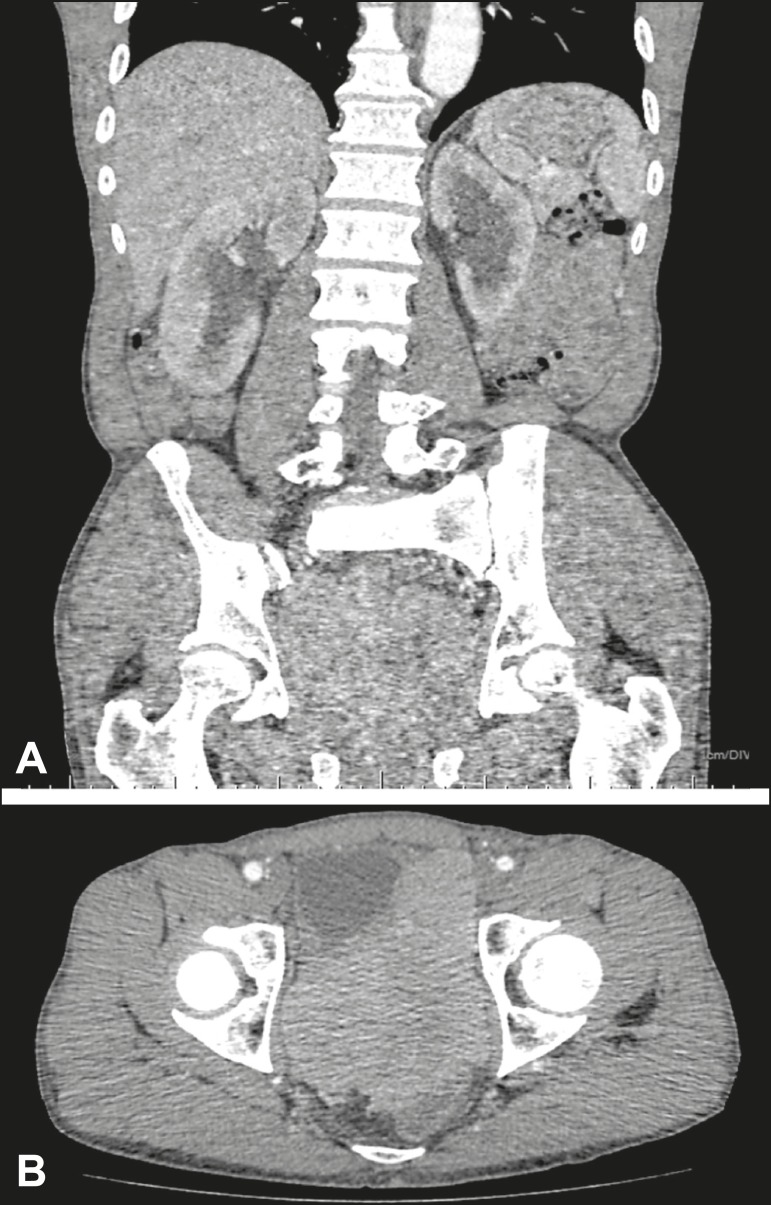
Contrast-enhanced coronal computed tomography (A) showing
